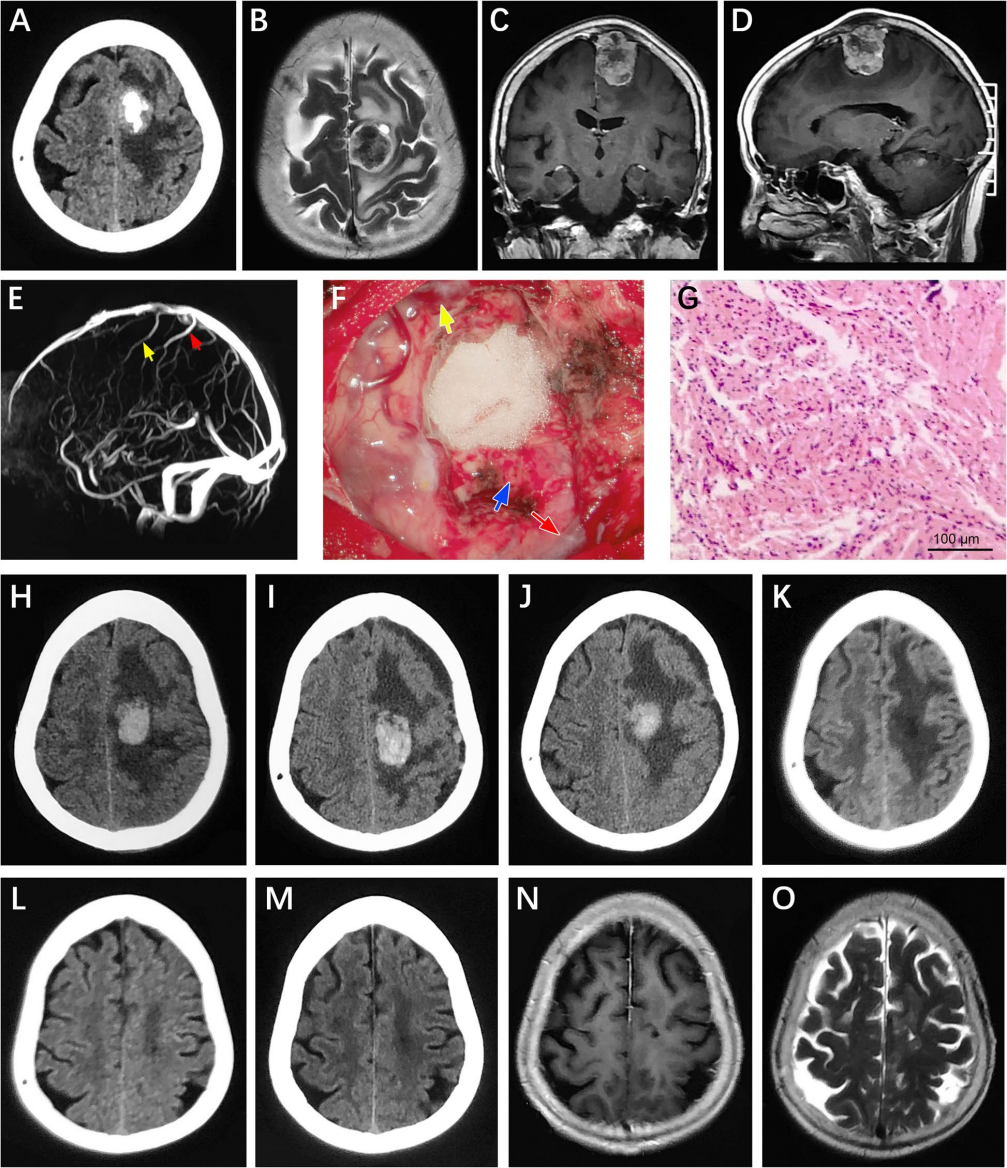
A CT showed intratumoral calcification. B Peritumoral edema was obvious. C-D The tumor invaded the skull. E MRV showed peritumoral vein accompanying (yellow and red arrows). F During the operation, the tumor was completely removed, peritumoral vein remained intact (yellow and red arrows), and the tumor invaded the pia mater and brain tissue (blue arrows). G Pathology showed atypical meningioma; H A small amount of hematoma in the operative area one day after operation, sober with right side muscle strength level 5. I Drowsiness with right side muscle strength level 3 three day after operation. J Lethargy with right side muscle strength level 1 five day after operation. K Drowsiness with right side muscle strength level 3 ten day after operation. L Sober with right side muscle strength level 4 fifteen day after operation. M Right side muscle strength level 4 one month after operation. N-O Right side muscle strength level 5 three month after operation
